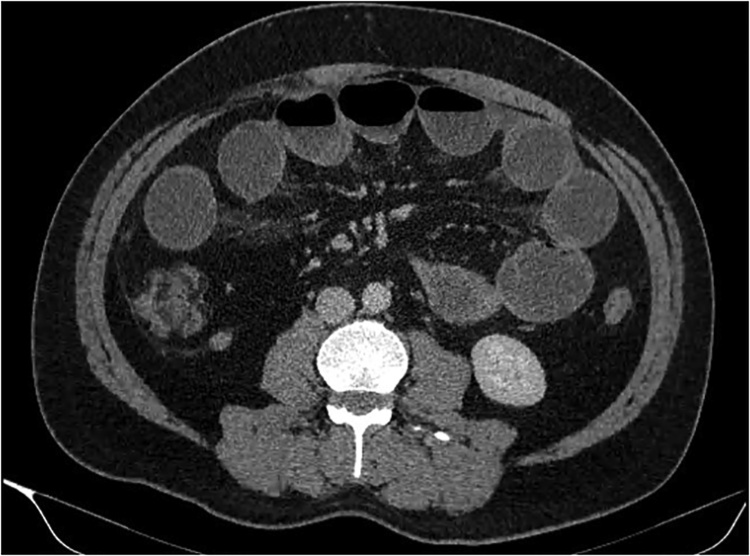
Axial view of the CT abdomen and pelvis showing multiple dilated small bowel loops consistent with SBO.
